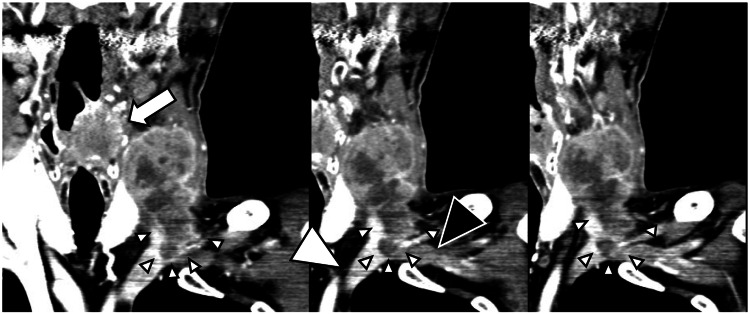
Preoperative post-contrast coronal CT images. A huge mass of metastatic lymph nodes is seen invading both the left internal jugular and subclavian veins (small arrow-heads). The left subclavian veins distal to the lesion (large black arrow-head; poor enhancement due to stagnant flow) and the left brachiocephalic vein (large white arrow-head; patent) are shown. A large primary hypopharyngeal tumor is visible (white arrow).
